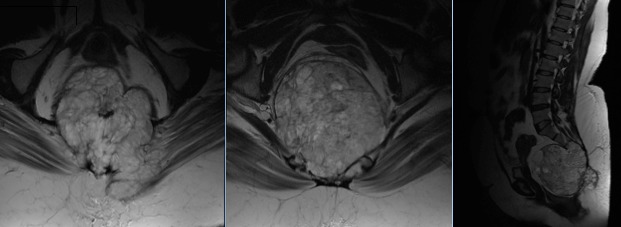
magnetic resonance imaging: large heterogeneous contrast-enhanced presacral mass with irregular contours, pushing the rectum, occupating the canal after lysis of the coccoyx, and involving the gluteal muscles
